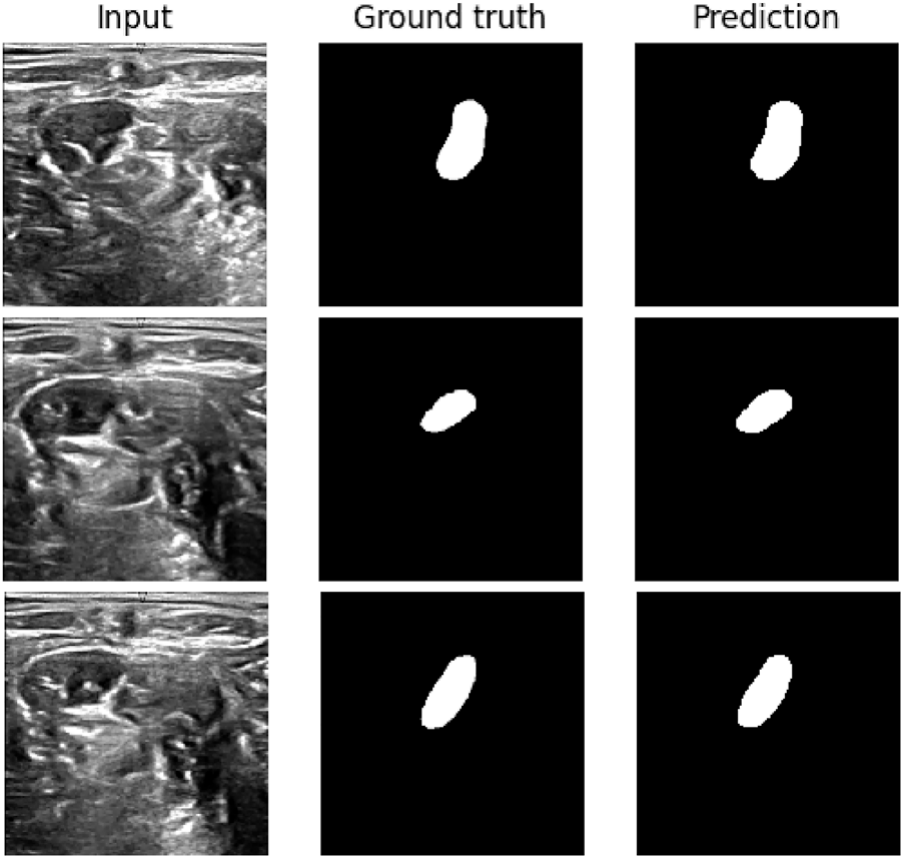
Ultrasound images of the neck as input on the left side, center shows the hand annotated nerve, right side shows the prediction by the model. These are some of the best results.
